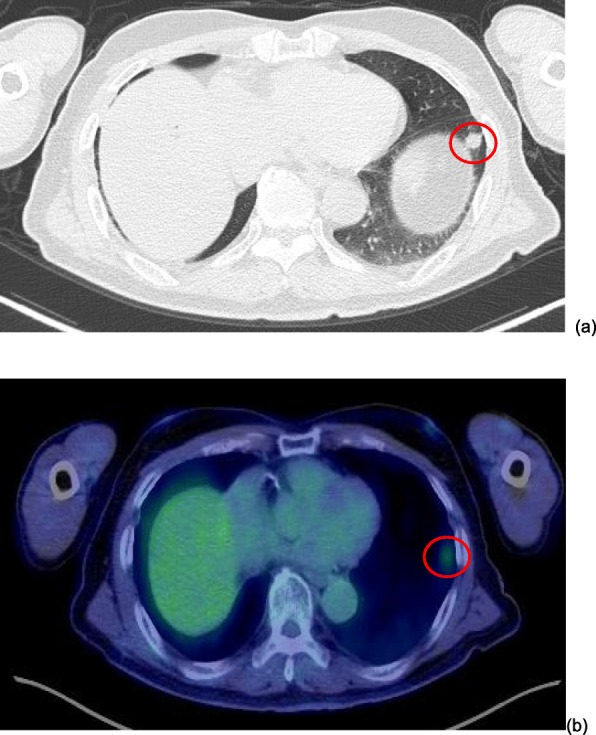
Computed tomography (CT) and positron emission tomography (PET) show a 10 × 10-mm tumor in the left lung S8 (a). This was a new isolated, localized, and solid lesion. Lung cancer metastasis was suspected but pleural indentation was also present, suggesting the possibility of primary lung cancer. PET shows strong uptake of fluorodeoxyglucose at the same site, suggesting lung metastasis (b)
